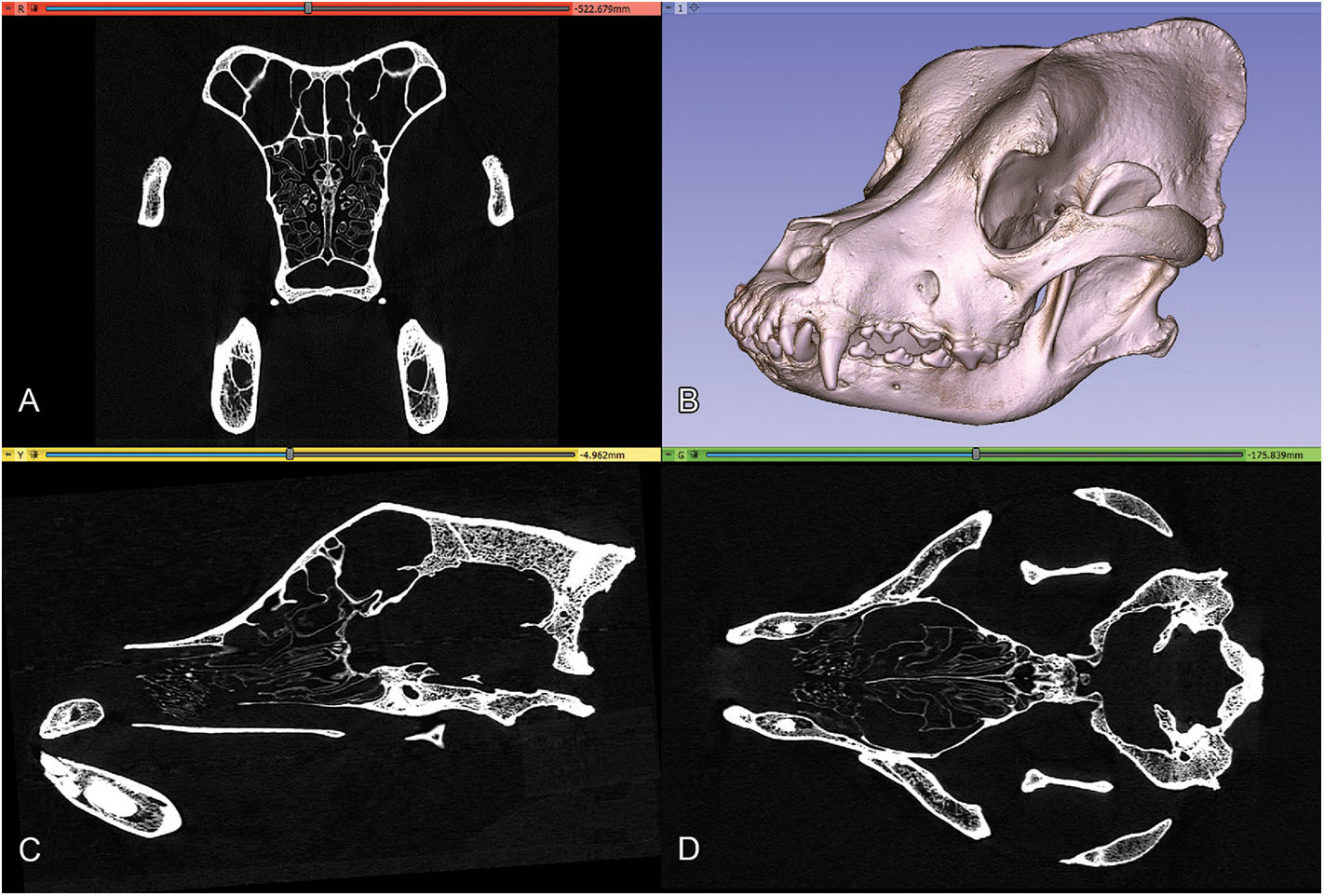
Orthogonal views and volume-rendered model of a Saint Bernard skull's CT series. (A) Transverse view. (B) 3D volume-rendered image, left rostro-lateral view. (C) Sagittal view. (D) Dorsal view.
